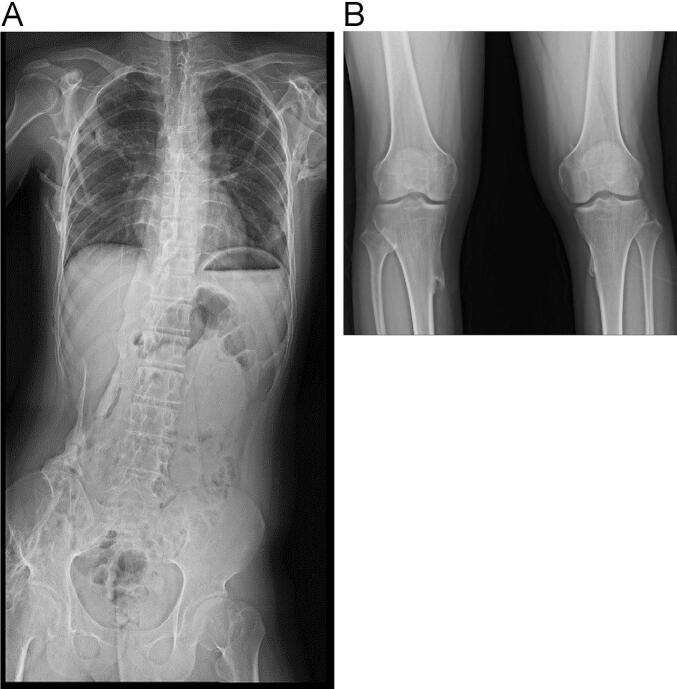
(A) Chest x-ray taken when the patient was in early adolescence. Heterotopic ossification was present in both shoulders and the chest, spine, and ilium. (B) Bony spur present at both proximal tibias.
